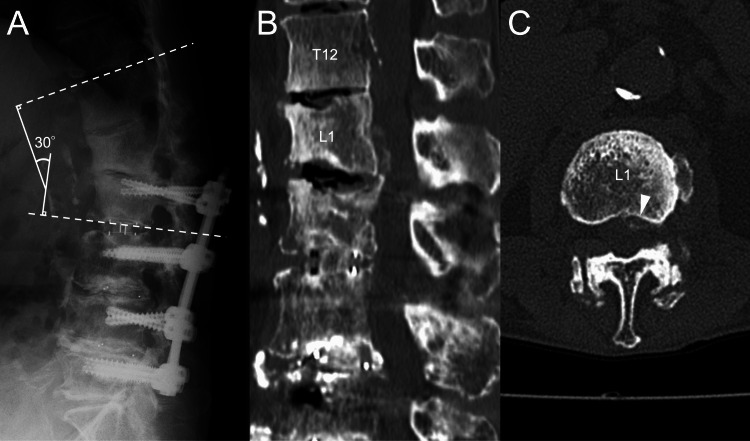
Image taken a year ago. (A) Lateral neutral radiographs show the disappearance of the disc space at L1-L2 and a kyphotic deformity (T12-L2 Cobb angle = 30°). (B) Sagittal CT image. (C) Axial CT image reveals a pale calcified lesion (indicated by white arrowhead) in the ventral epidural space at the L1 level.
